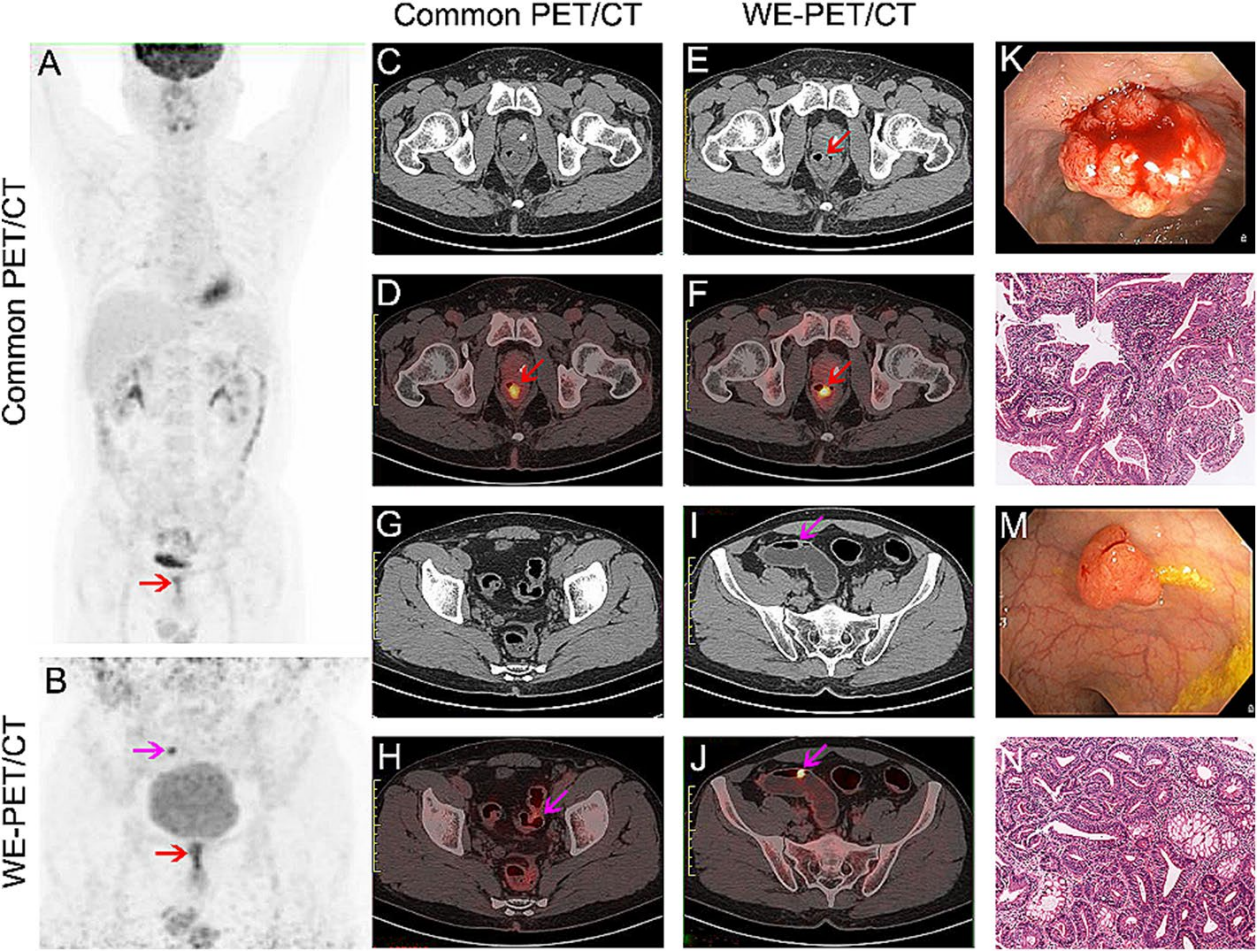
A 46-year-old male patient undergoing cancer screening. One incidental focal ^18^F-FDG uptake in the rectum was observed on common PET/CT (A, C-D). Two intraluminal nodules with ^18^F-FDG uptake in the rectum and sigmoid were detected on WE-PET/CT (B, E-F and I-J). The colonoscopy and pathological analysis confirmed tubulovillous adenoma of the rectum (K, L) and tubular adenoma with high-grade intraepithelial neoplasias of the sigmoid (M, N)
